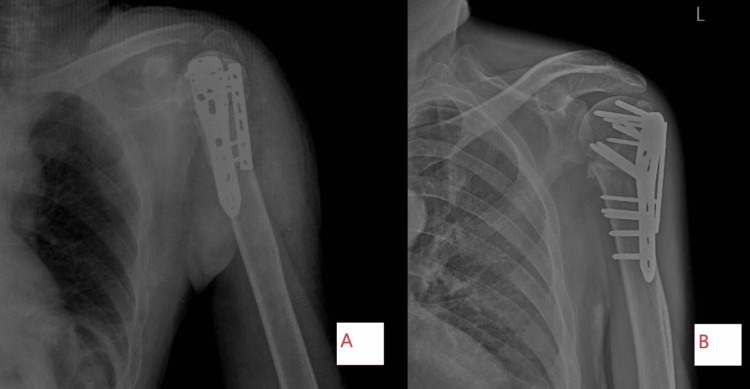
Post-operative plain radiograph antero-posterior and lateral view of dual plating for proximal humerus fracture. (A) Plain radiograph lateral view of left shoulder. (B) Plain radiograph antero-posterior view of left shoulder.
